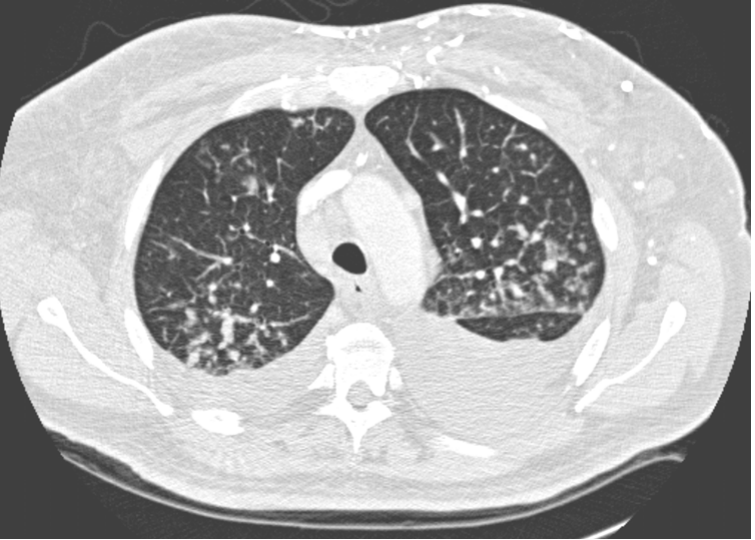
Axial CT of the mid thorax with lung window settings: bilateral pleural effusions and diffuse septal thickening consistent with lymphangitis carcinomatosa.
